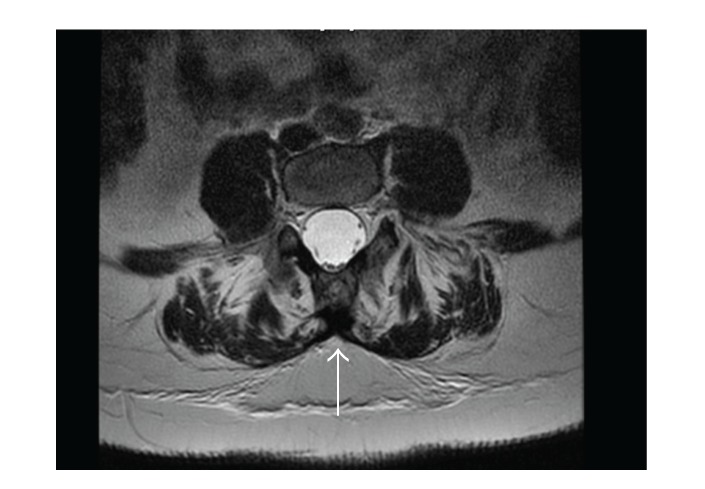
The patient's MRI transversal view: evidence of sacral bone interruption.
